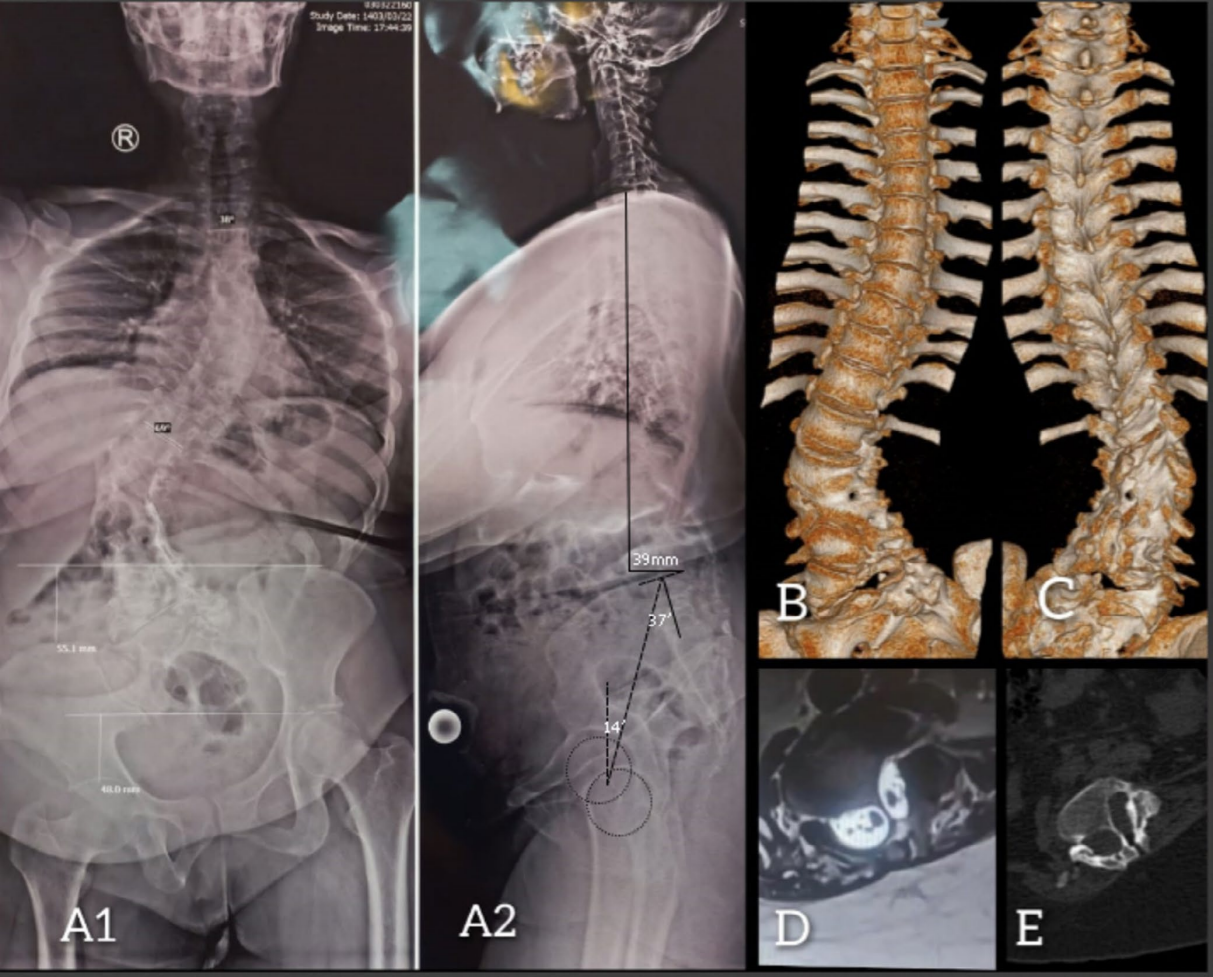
(A) 36 in. scoliosis radiography shows 70° scoliosis in thoracolumbar region. A1 coronal view: Shoulders height difference is 26 mm, pelvic obliquity about 55 mm, and femoral head height difference is 48 mm. Left side waist crease is prominent. A2 Sagittal view: Acceptable sagittal vertical alignment without compensatory pelvic retroversion. (B) and (C) show Anterior and posterior view of the 3D reconstructed CT scan of the spine. (D) and (E) shows type 1 split cord malformation (SCM).
